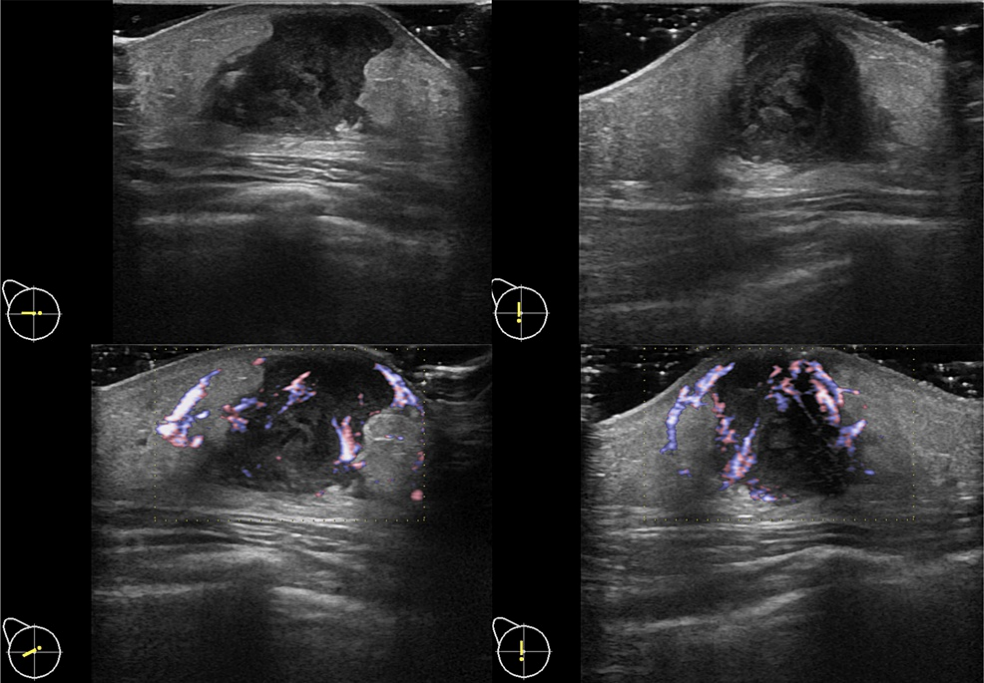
Ultrasonography showed a 26 mm × 18 mm irregularly shaped heterogeneous low echo-level area, poorly marginated, with a high blood flow signal suggesting a breast abscess.
